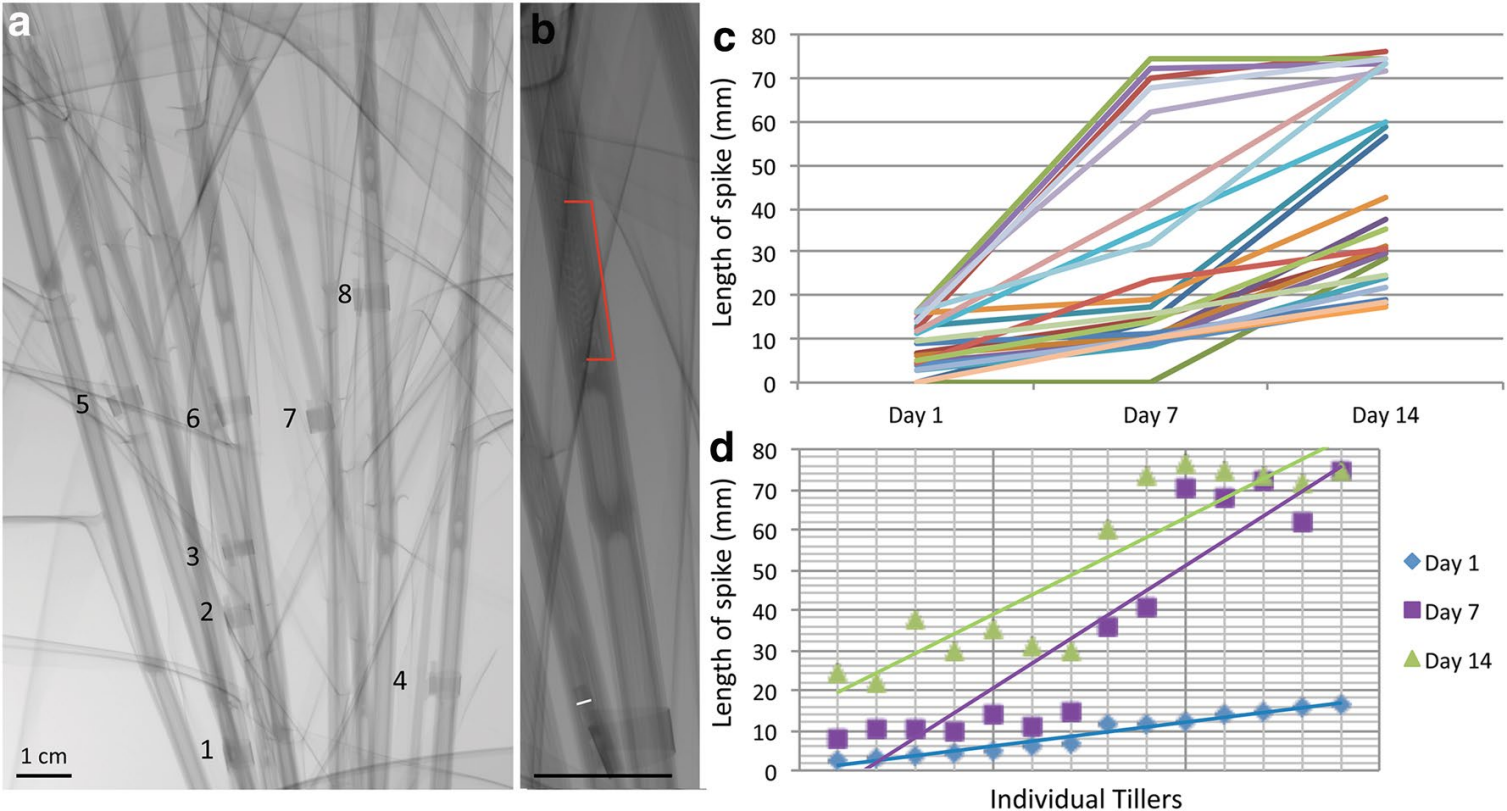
In planta* radiograph image of Barley and spike size analysis. a Whole plant image slice of Barley, showing 8 tagged tillers. b Close up image of the whole Barley showing floral spike size highlighted by *red segment* and *white line* represents fixed marker (1.2 mm). *Scale bar* indicates 1 cm. c
